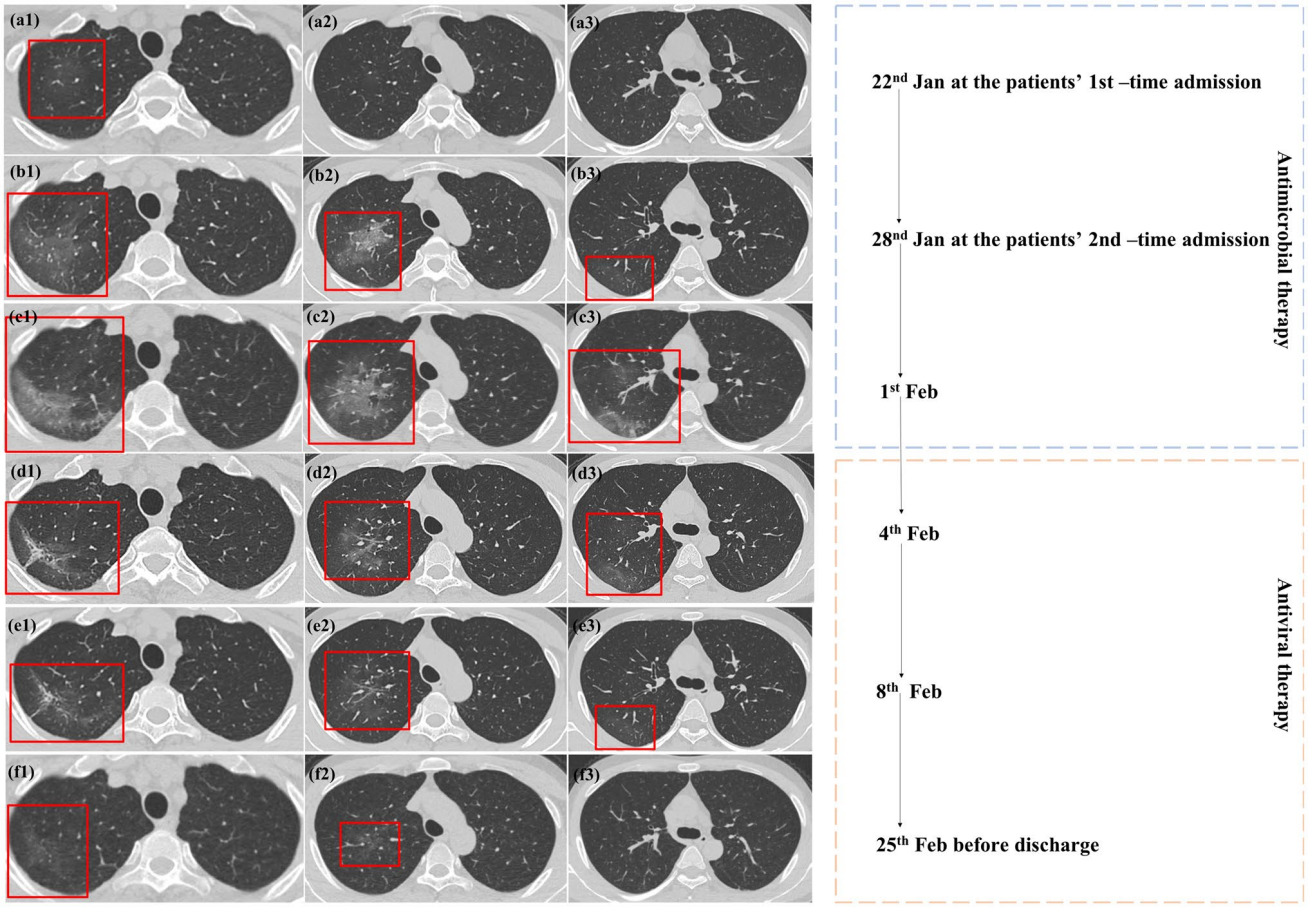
Comparison between the initial and follow-up CT scans. a1–a3 were on 22nd Jan at the patient’s 1st-time admission. b1–b3 were on 28th Jan at the patient’s 2nd-time admission with the same level of a1–a3 separatory. c1–c3 were on 1st Feb after antimicrobial therapy. d1–f3 were CT scans during antiviral therapy. a1 showed lightly ground-glass opacity (GGO) located at the right apical segment, which was prominently enlarged on b1 and b2, b3 showed newly increased GGO, as well as evidence of fibrosis (b2). c1–c3 showed enlarged GGO and fibrosis after antimicrobial therapy. d1 shows newly increased fibrosis, which was reduced in e1 and disappeared in f1. d1–f3 showed that the lesions gradually decreased after antiviral therapy
